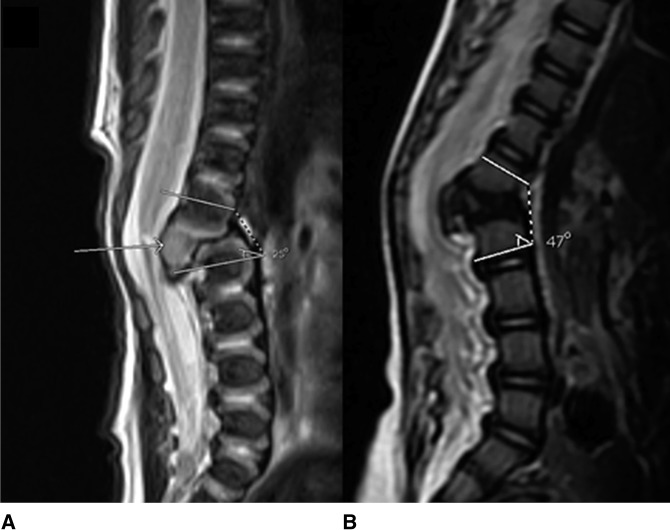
A, MRI at day 2 of life shows notable canal intrusion (arrow) and 25° local kyphosis. B, MRI at 9 months of age shows 47° of local kyphosis. Both are done supine, under anesthesia. (Reproduced with permission from the Children's Orthopaedic Center, Los Angeles, CA.)
